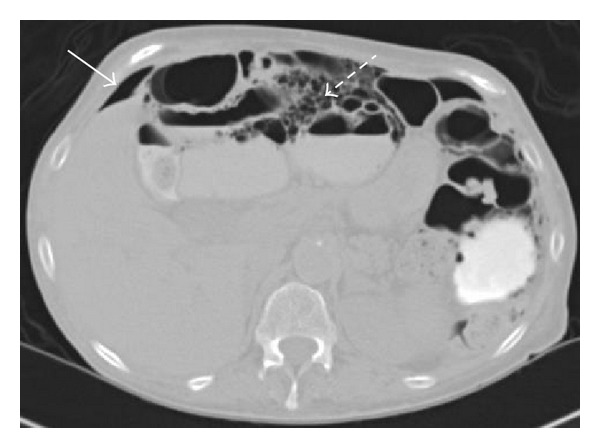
Axial CT image with enteric contrast demonstrates pneumoperitoneum in the right upper quadrant of the abdomen, anterior to the liver (arrow). Multiple large “cysts” are seen within the wall of the small bowel (dashed arrow), consistent with “pneumatosis cystoides intestinalis” seen in this patient with scleroderma. Contrast is noted in a loop of small bowel at the left side, without extravasation of contrast from the small bowel.
